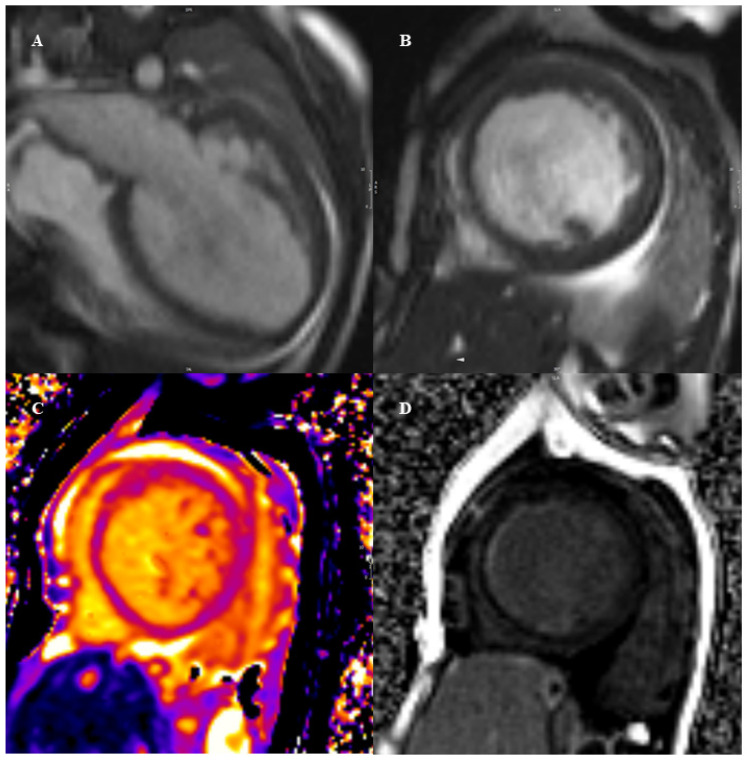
A case of DCM in a 6-year-old child, male. Cine sequences documented diffuse hypokinesia, septal dyssynergy and severe left ventricular dilatation (left ventricular end diastolic volume indexed 158 mL/m^2^) with a severely compromised ejection fraction (LVEF 12%) as shown in panels (A,B). The T2-mapping sequences showed diffusely elevated T2 values (72–75 ms), as a sign of concomitant myocardial edema (panel (C)), which was not evident in T2-weighted imaging. Late gadolinium enhancement was suggestive for septal mid-wall hyperenhancement (panel (D)).
